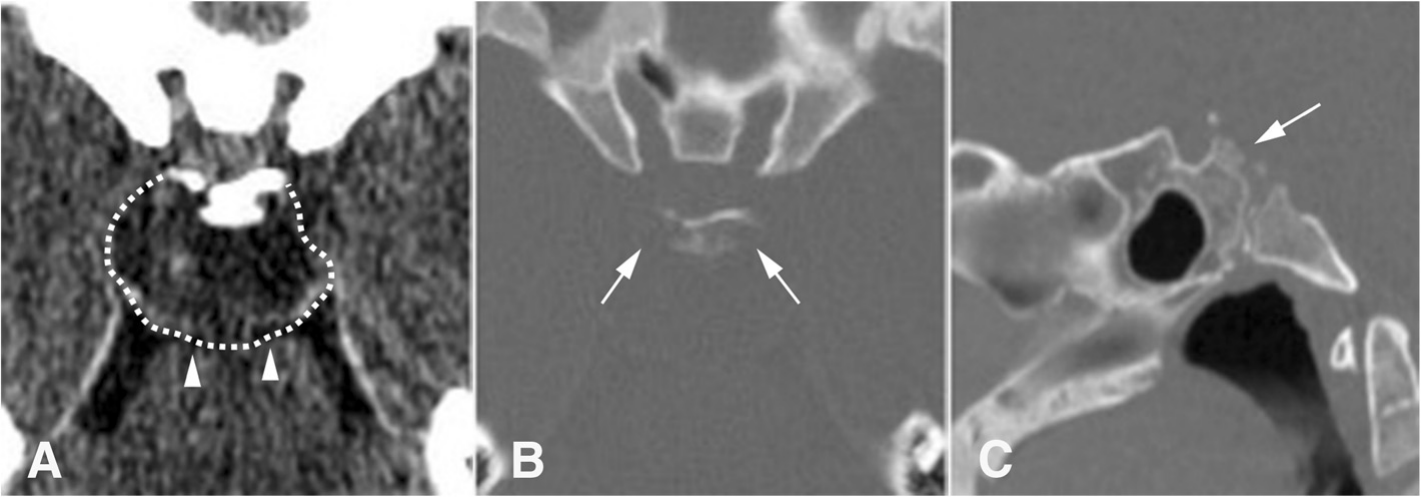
CT scanner of a clival chordoma. a. Axial brain window. The retroclival lesion developed posteriorly (dotted line) with contact to the brainstem (arrowhead). b. Axial bone window. Erosion of both posterior clinoid proccesses by the chordoma (arrows). c. Erosion of the posterior side f the clivus (arrow)
